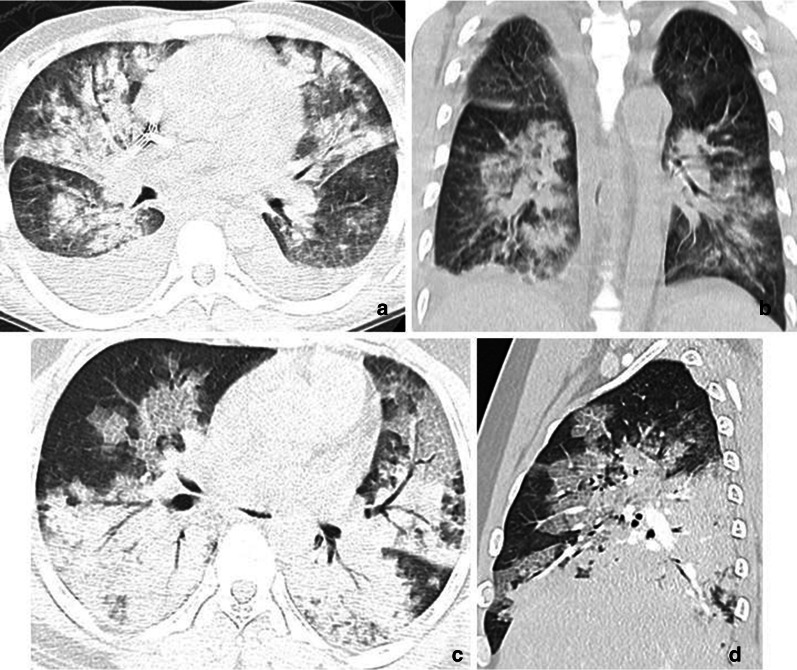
Non-infectious mimickers of indeterminate COVID-19. a, b A 73-year-old man with pulmonary edema, axial and coronal reformatted CT images show bilateral perihilar consolidation and to less extent GGO associated with bilateral pleural effusion and pericardial effusion in mediastinal window (not shown). c, d A 58-year-old woman with acute respiratory distress syndrome, axial and sagittal reformatted CT images shows dependent consolidation and multifocal non-rounded GOO superimposed with interlobular septal thickening (crazy paving)
